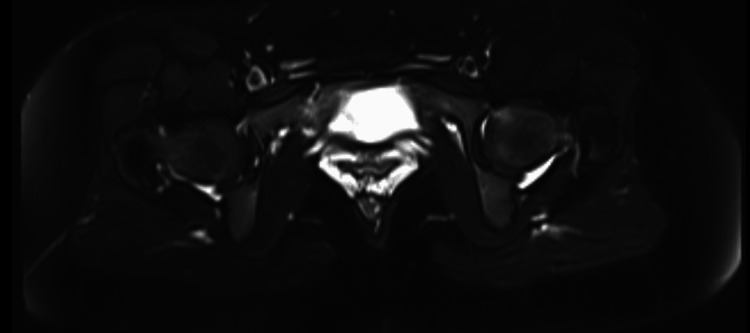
Post-operative contrast-enhanced pelvic MRI T1 axial
